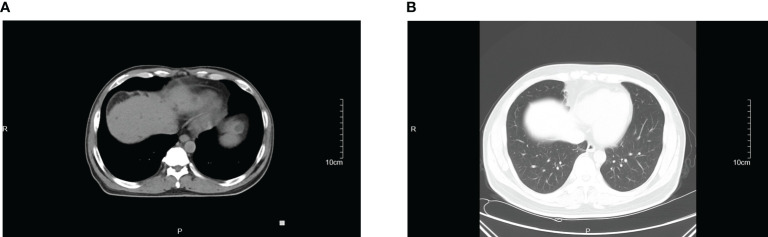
The CT images of abdomen (A) and lung (B) after treatment.
